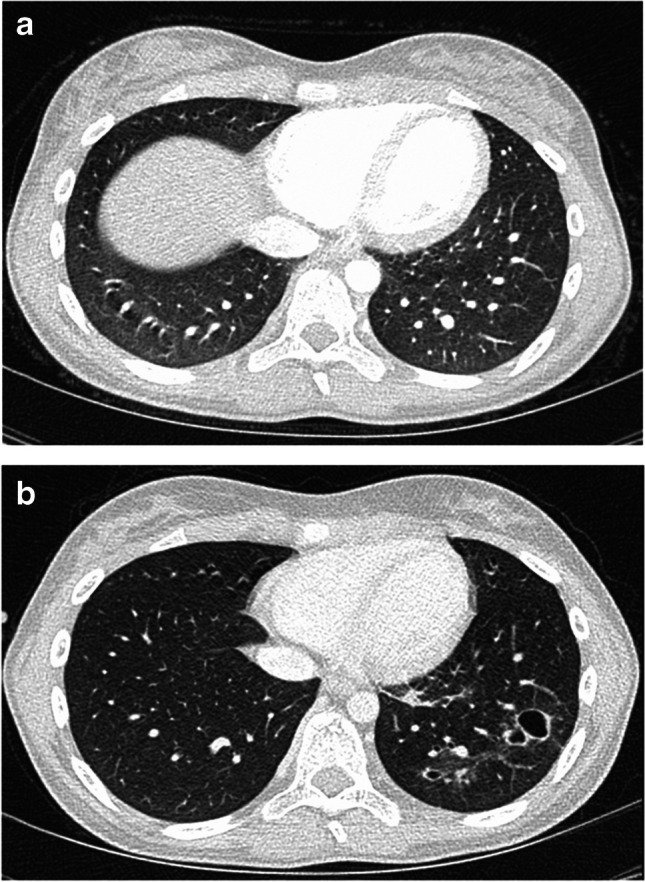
Contrast-enhanced axial computed tomography scans on lung windows in a 13-year-old girl with Hodgkin lymphoma. a Initial staging. b Early response assessment with newly developed cavitation in the left lower lobe caused by fungal infection facilitated by immunodeficiency
